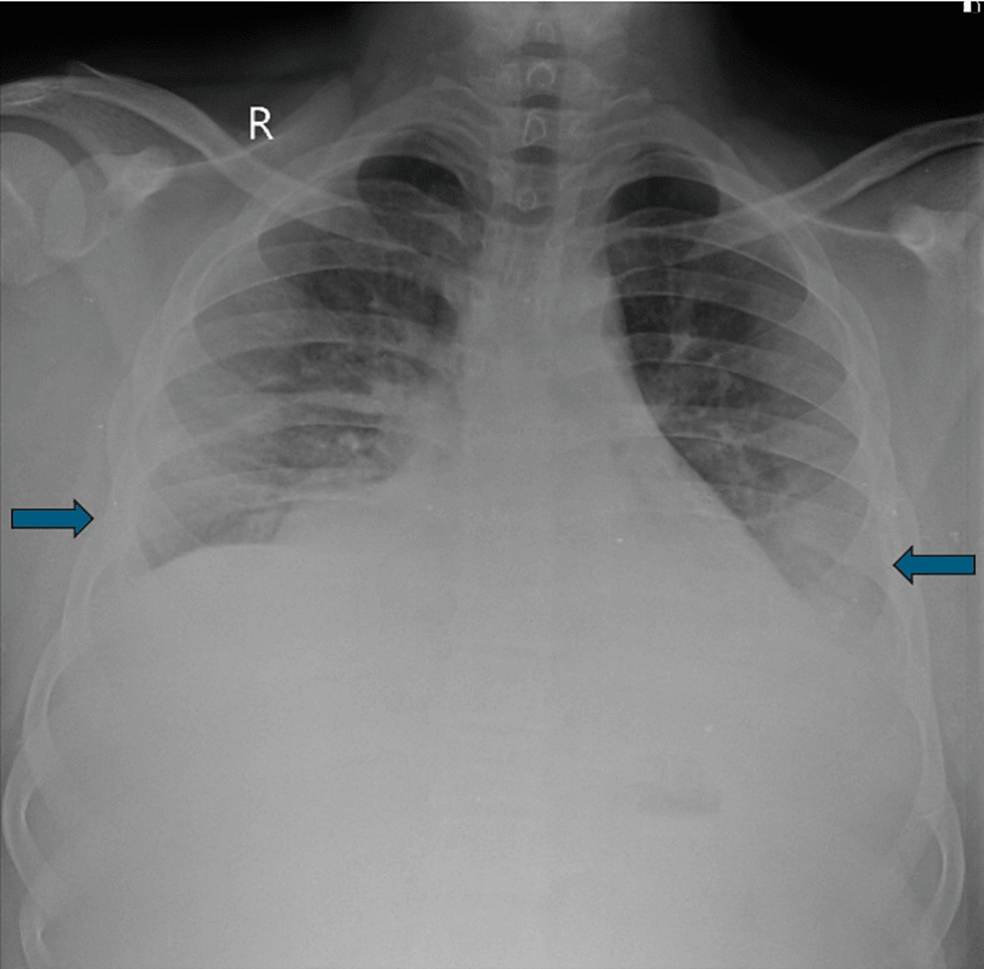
Chest radiograph of the patient showing bilateral pleural effusion denoted by the blue arrows.
